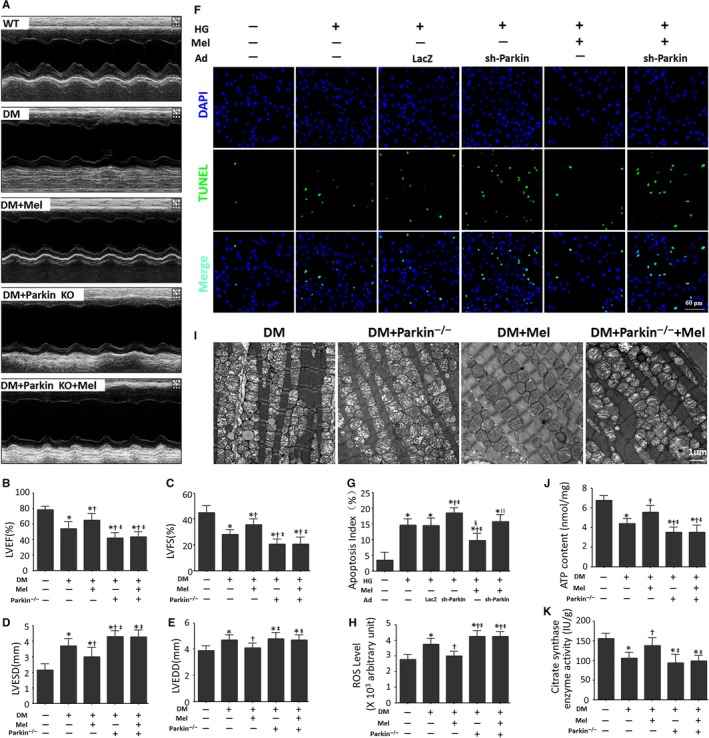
Melatonin ameliorates DCM phenotypes via Parkin. A, Representative images of echocardiography. B, Measurements of LVEF (%). C, Measurements of LVFS (%). D, Measurements of LVESD (mm). E, Measurements of LVEDD (mm). The columns and error bars represent the means and standard deviations (SD) (n = 12). P* < 0.05 vs WT; ^†^
